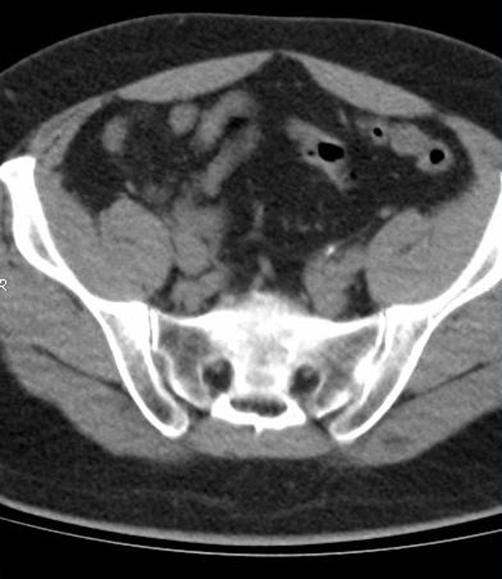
Abdominal CT revealing a 9-mm hyperdense
